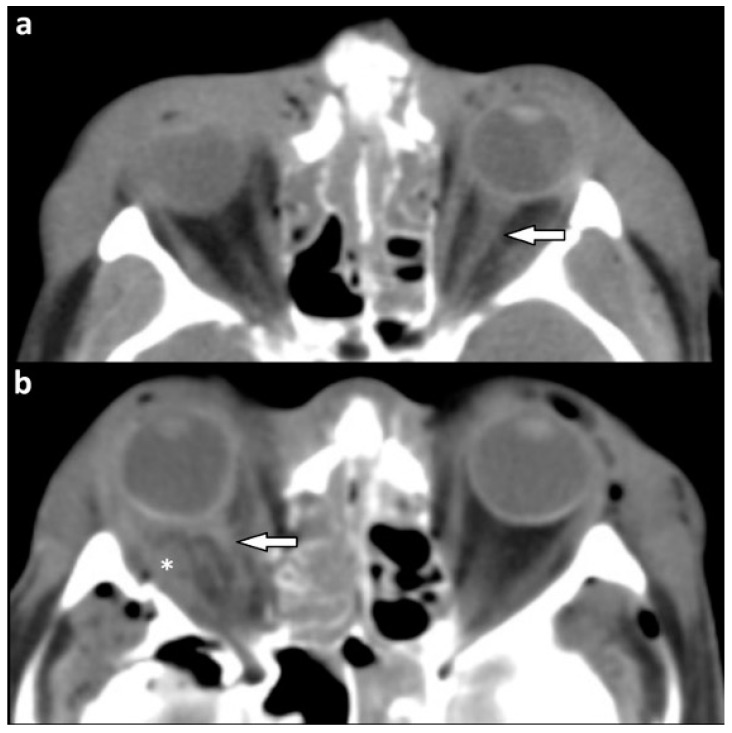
Elongated optic nerves associated with traction trauma. NE-MSCT, soft tissue window, axial plane. (a) The left optic nerve (white arrow) appears elongated and hyperdense due to intraneural bleeding. (b) Swelling and deformation of the right optic nerve (white arrow) and retrobulbar hematoma (asterisk) in a complex midface fracture involving all orbital walls (NE-MSCT = non-contrast-enhanced multi-slice CT).
